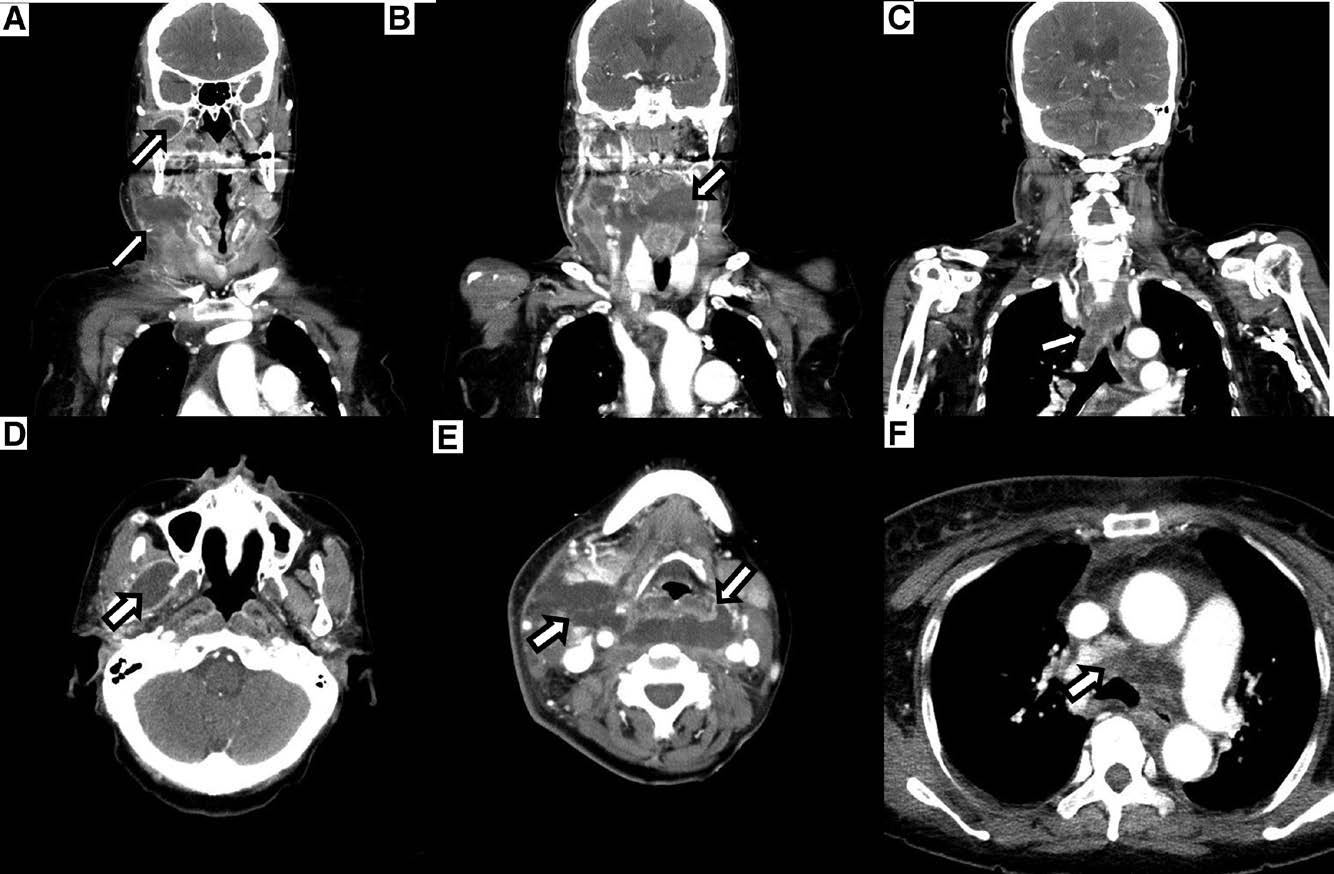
Neck CT with intravenous contrast. (A–C) Coronal view of CT show abscess formation from the right maxillary sinus to mediastinal space. (D–F) Horizontal view of CT shows abscess formation from right maxillary sinus to mediastinal space as same as coronal view. CT = computed tomography.
